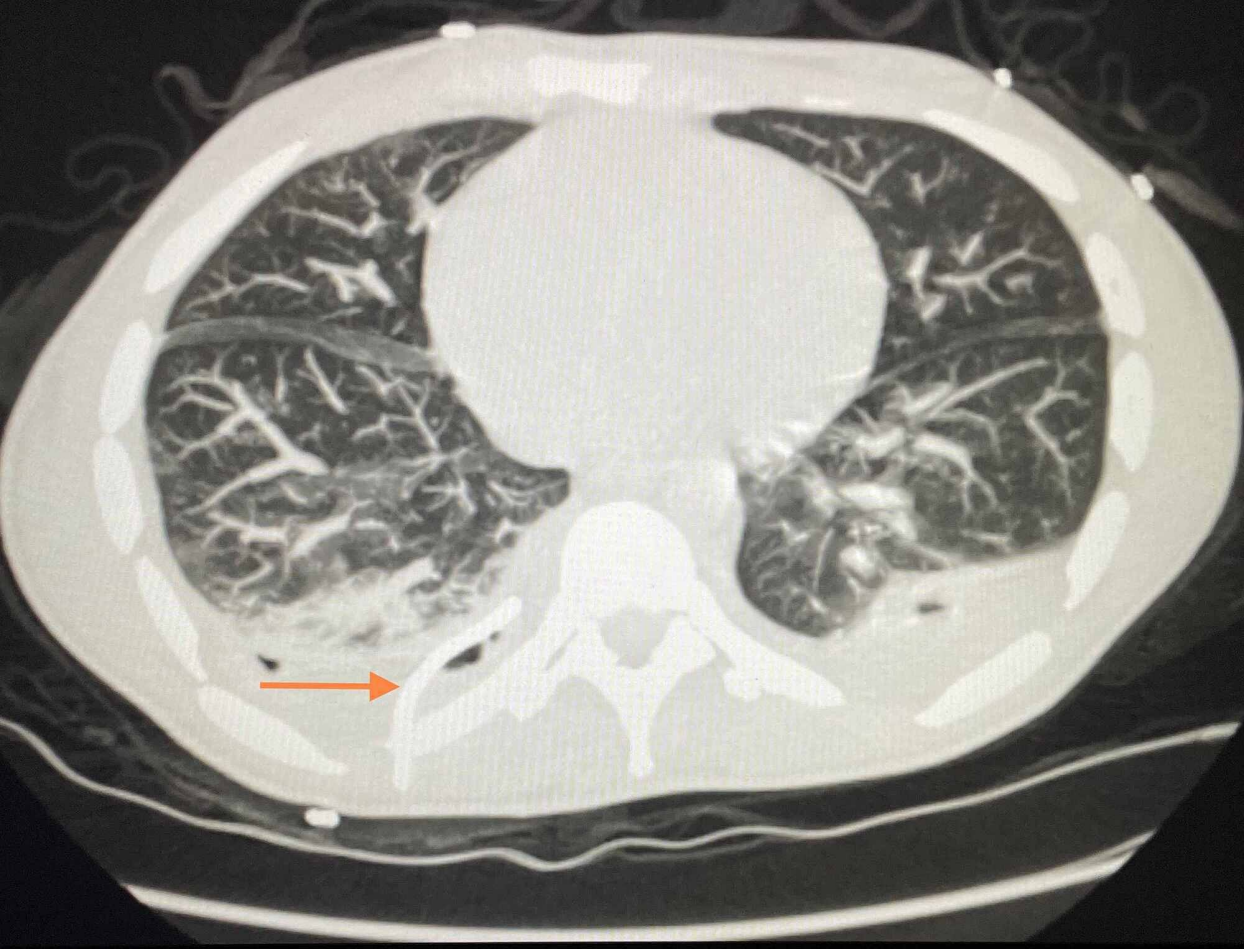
Reformatted CT chest without contrast and with pigtail catheter. Pigtail catheter (orange arrow) and resolving pleural effusion on the left following TPA administration. TPA, tissue plasminogen activator
